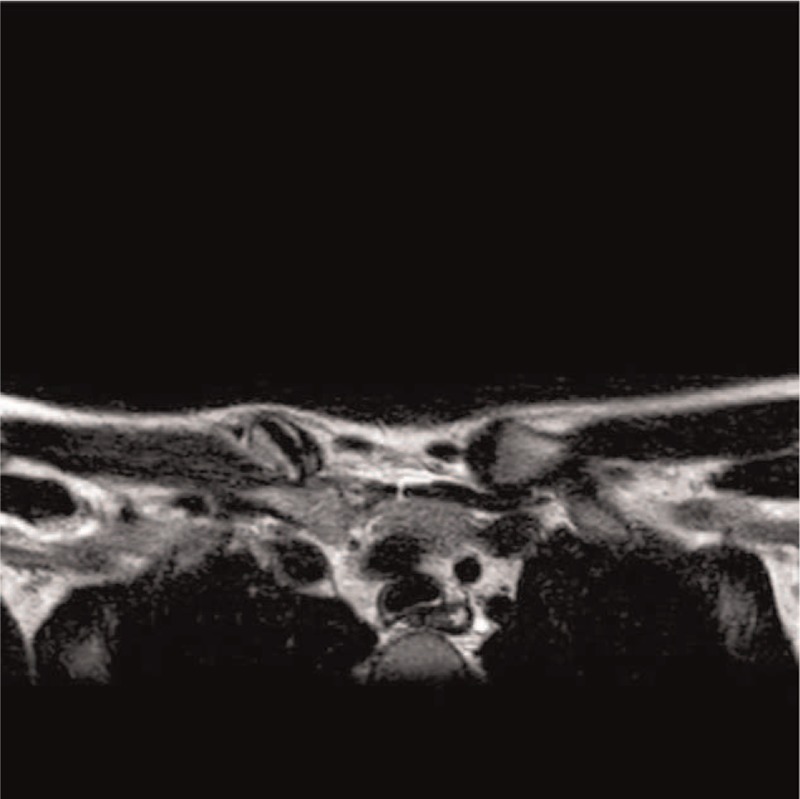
Magnetic resonance imaging showing a clavicular Salter-Harris type 2 fracture.
